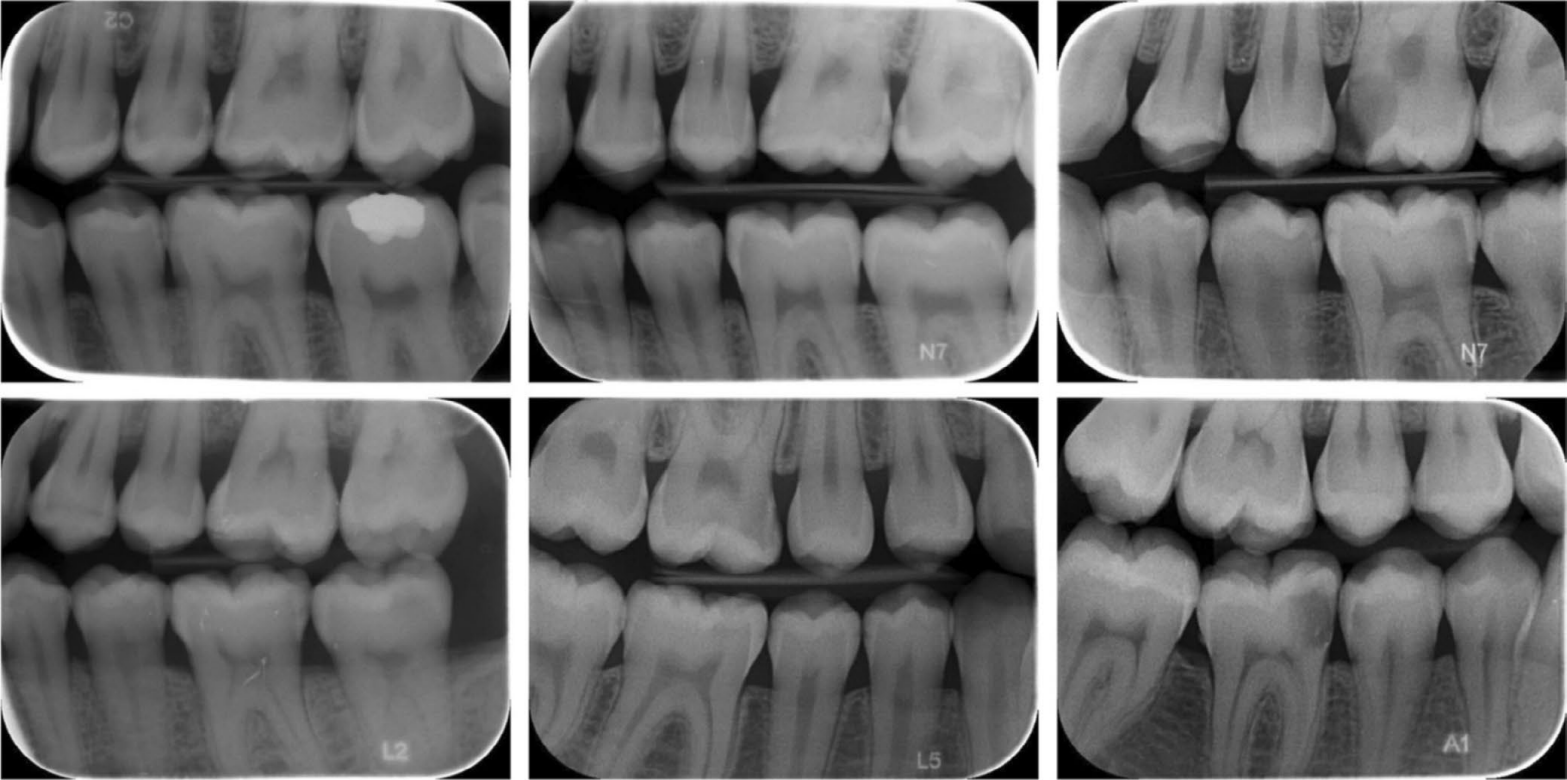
Bitewing samples from dataset.
